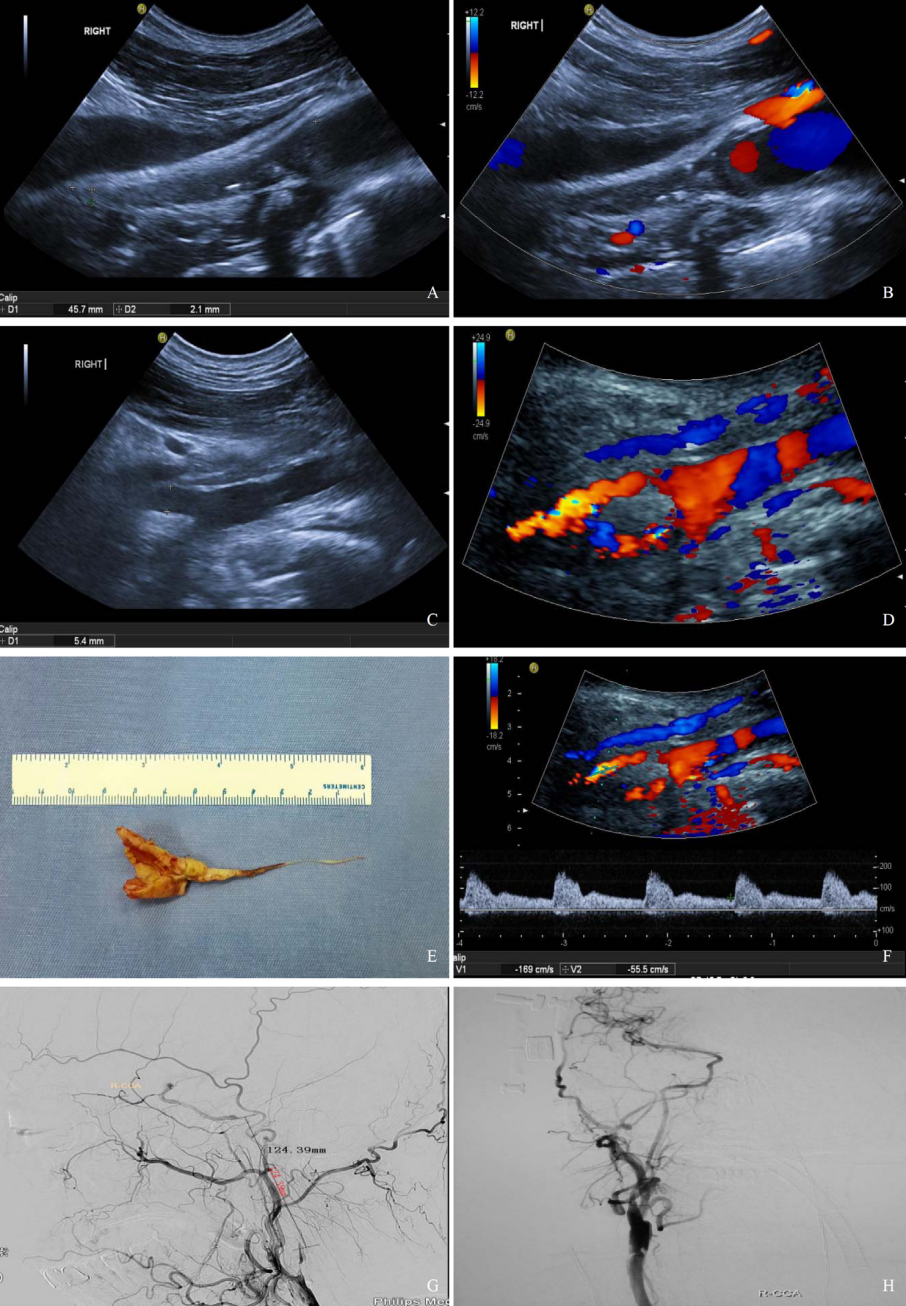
A 60-year-old male patient with right ICA occlusion underwent CEA. (A) Before CEA, two-dimensional ultrasonography image showing that the right ICA was filled with a hypoechoic lesion. For the original occlusion, the distal diameter was 2.1mm and the length of occlusion in the ultrasonic visual range was 45.7mm. (B) Color Doppler image showing no flow in the right ICA before CEA. (C) Two-dimensional ultrasonography image showing that the right ICA was reopened after CEA. The proximal diameter was 5.4mm. (D) Color Doppler image showing the blood flow filled in the right ICA after CEA. (E) The plaque and clot. (F) After CEA, the spectral wave form showing the peak systolic velocity of right ICA was 169cm/s, and the end diastolic velocity was 55.5cm/s. (G) Before CEA, DSA image showing occlusion of the right ICA and blood retrograde flow into the cavernous portion of the ICA. The occlusion length was 124.68mm. (H) After CEA, DSA image showing the right ICA was repatency.
